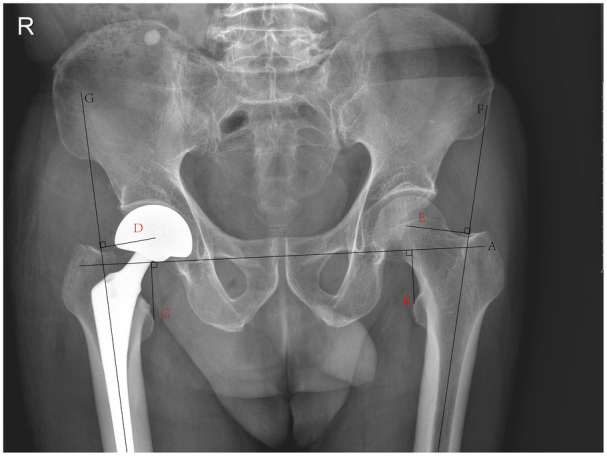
Postoperative anteroposterior pelvic radiograph demonstrating radiographic precision of component positioning [horizontal femoral offset (HFO) and leg length discrepancy (LLD)]. A, inter-teardrop line; B, distance from the most prominent point of the lesser trochanter on the healthy side to line A; C, distance from the most prominent point of the lesser trochanter on the operated side to line A; D, offset on the operated side; E, offset on the healthy side; G, femoral shaft bisector line on the operated side; F, femoral shaft bisector line on the healthy side.
